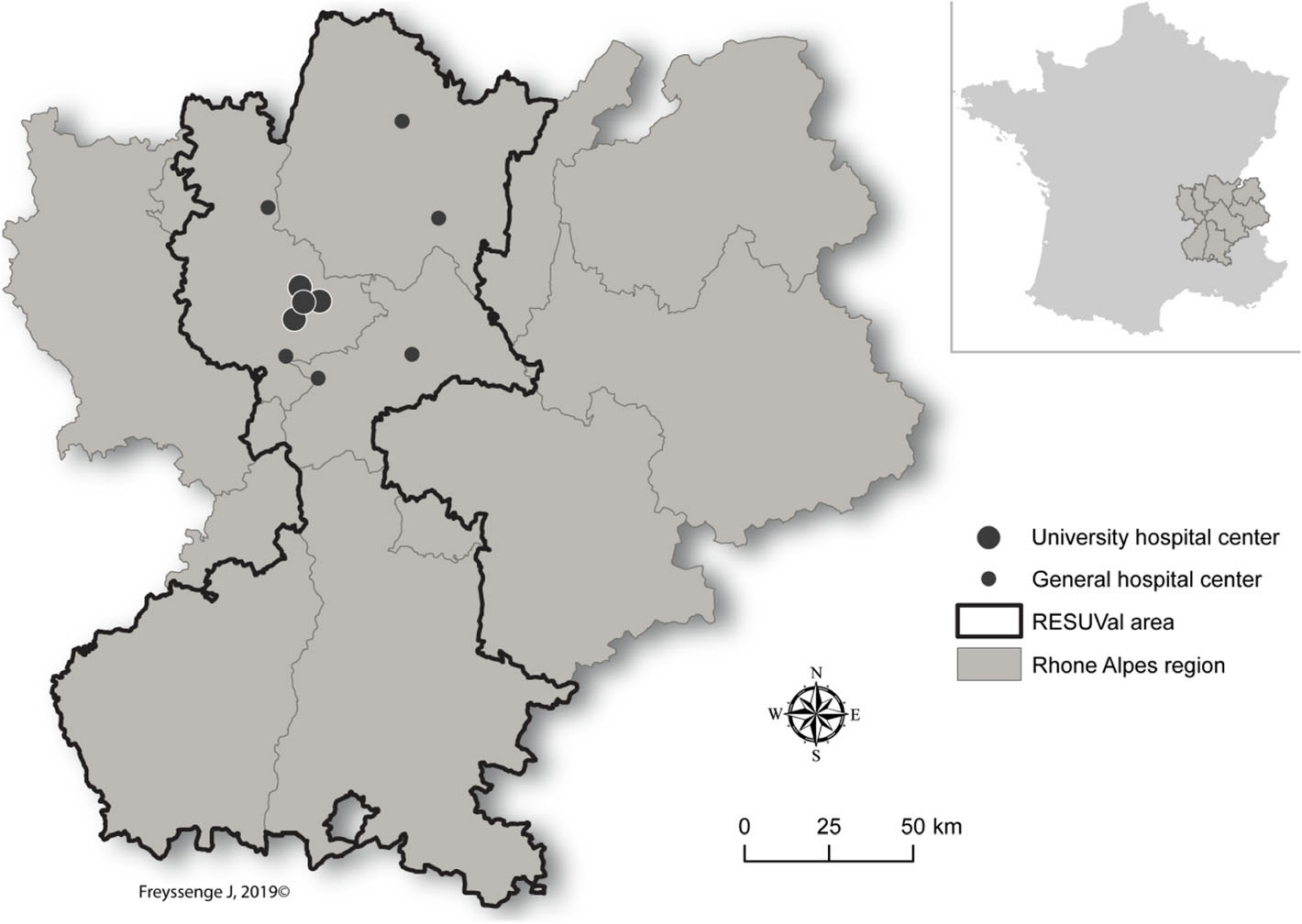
Geographic area of the RESUVal network
